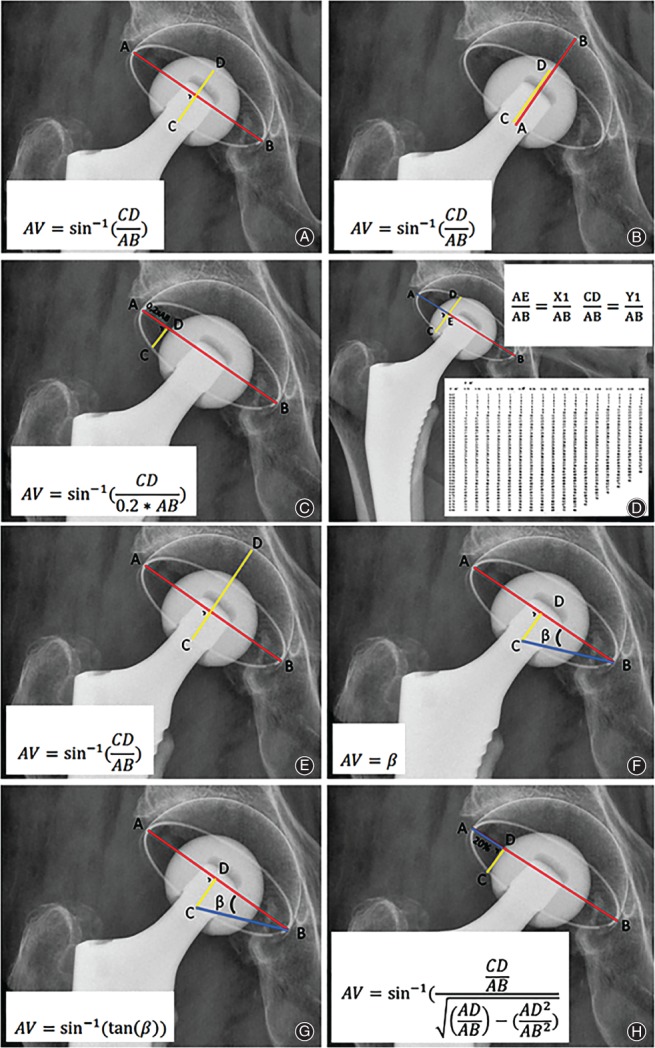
Category 3 methods. Definitions and algorithms of the included anteversion (AV) measurements methods with anterior–posterior radiographs. The respective distances are measured and filled in the respective formulas: (A) Lewinnek *et al.*
